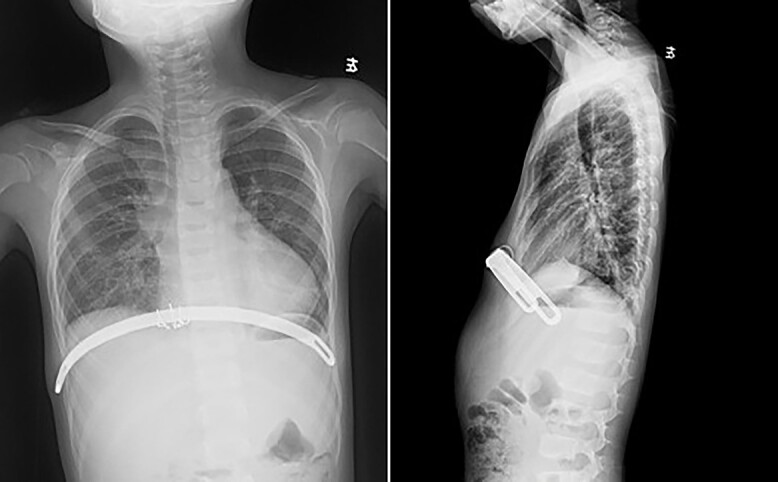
Chest X-ray examination after the first operation.
